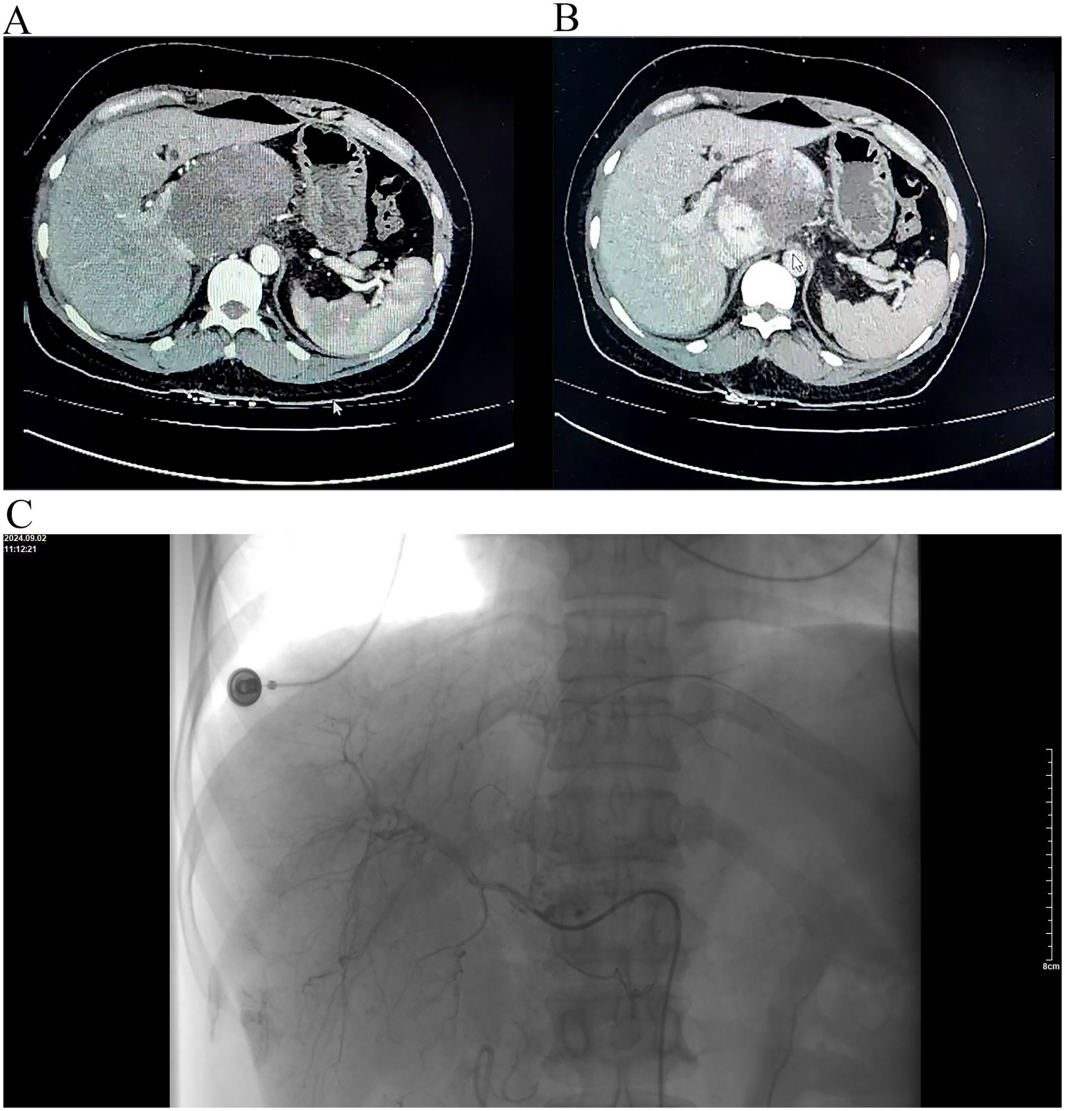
(A) Arterial phase of contrast-enhanced CT demonstrates a giant hemangioma in the caudate lobe. No hepatic capsular abnormality is evident. observed. (B) Venous phase of contrast-enhanced CT. (C) Hepatic arteriography confirms the presence of the hemangioma; however, no definite feeding vessel or subcapsular hematoma is identified.
